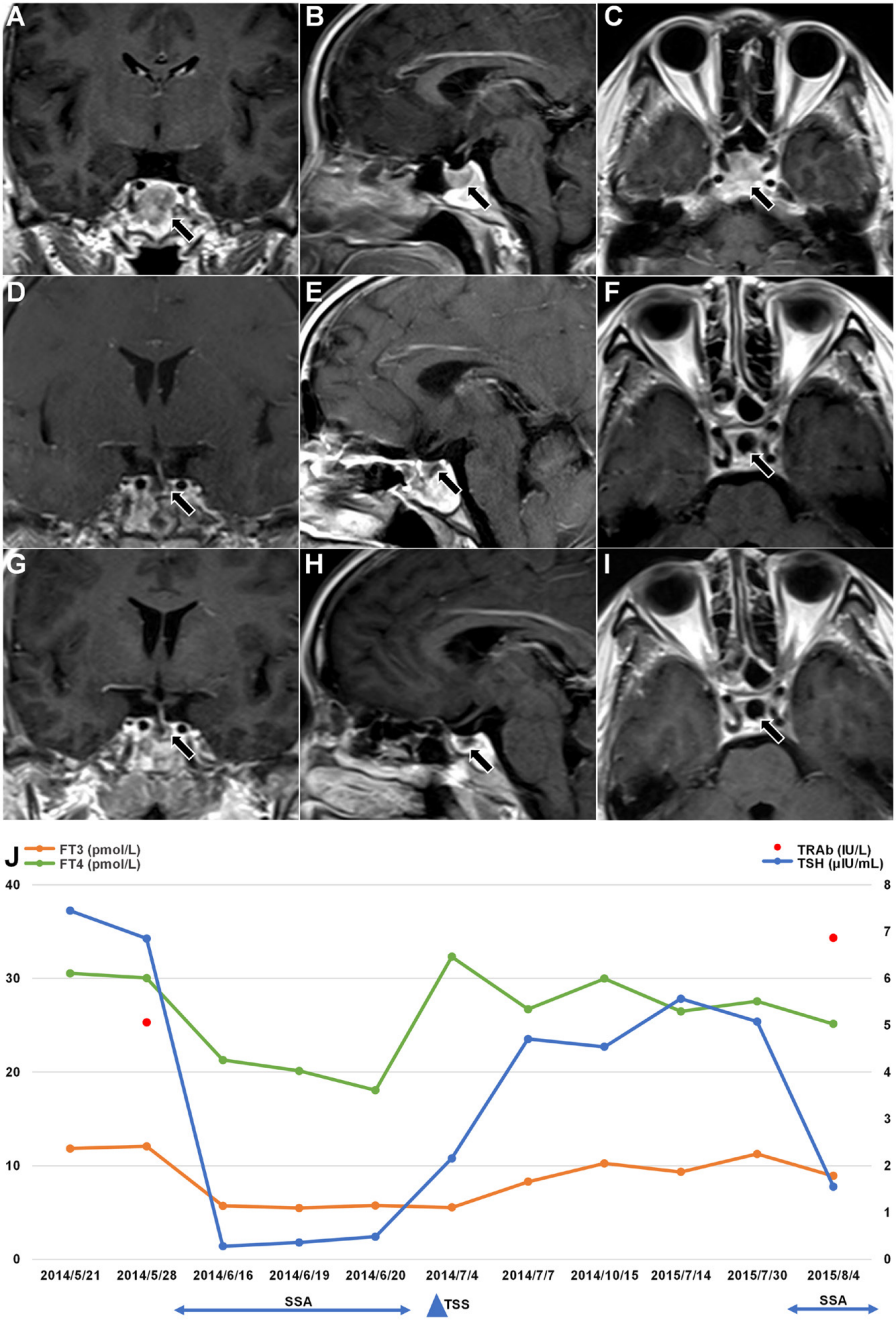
Pituitary enhanced MRI, thyroid function change, and treatment of case 2. FT3, free tri-iodothyronine; FT4, free thyroxine; SSA, somatostatin analog; TRAb, thyroid-stimulating hormone receptor antibodies; TSH, thyroid-stimulating hormone; TSS, transsphenoidal surgery. Normal ranges: FT4 7.64–16.03 pmol/L, FT3 3.28–6.47 pmol/L, TSH 0.49–4.91 μIU/mL, TRAb 0–1.7 IU/L.
